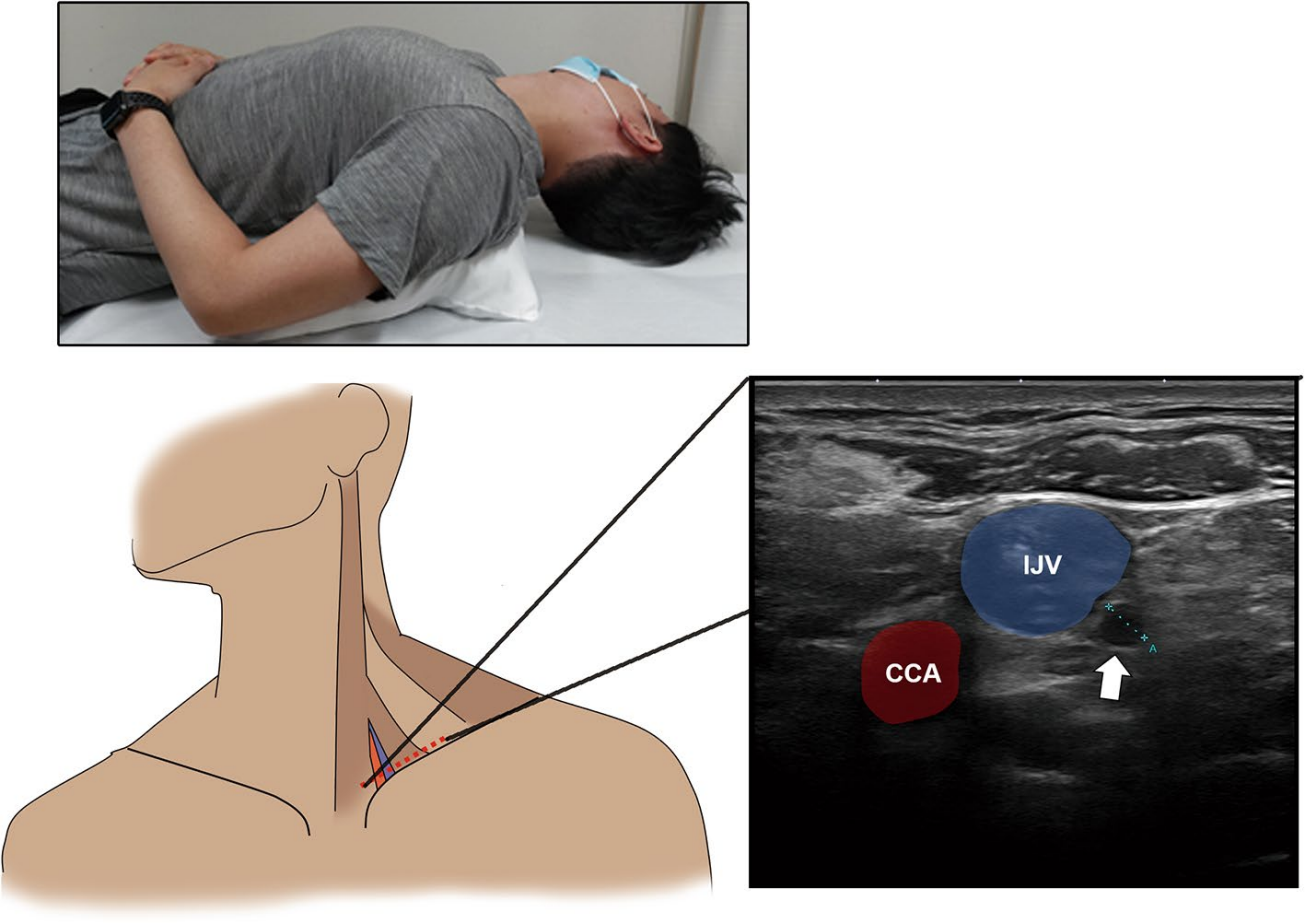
Participant position for the sonography examination. Participants are positioned supine with their chin slightly raised, face tilted approximately 30° to the right side, and with a pillow inserted under the scapula for support. The sonographic probe is positioned perpendicular to the skin and parallel to the clavicle (). The sonographic image shows the short axes of the CCA (), IJV (), and TD ().
